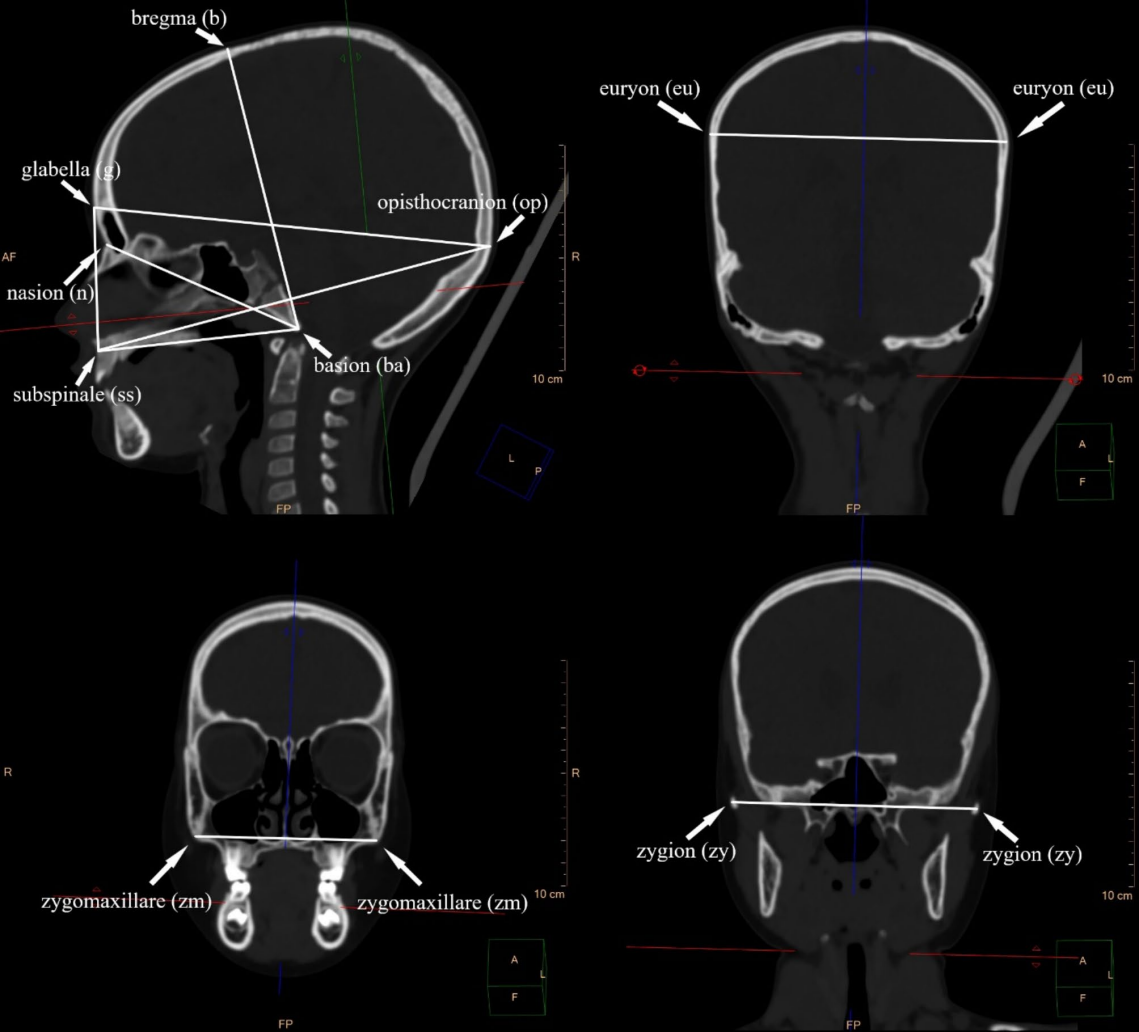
Computed tomography of the head in children demonstrating cranial landmarks and measurements cranium used in the study.
